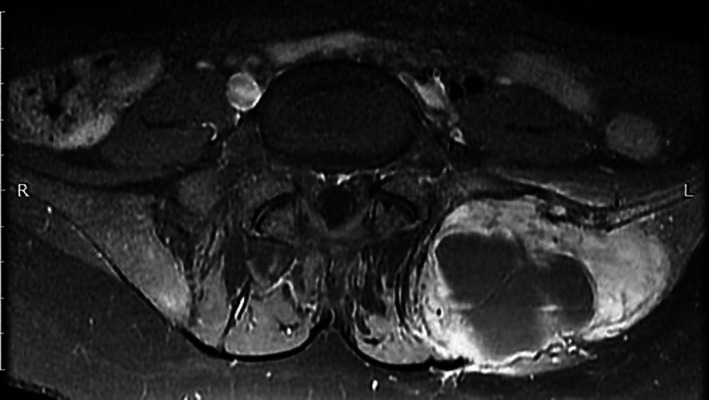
MRI showing a brown tumor of the left iliac bone of a 24‐year‐old woman.
